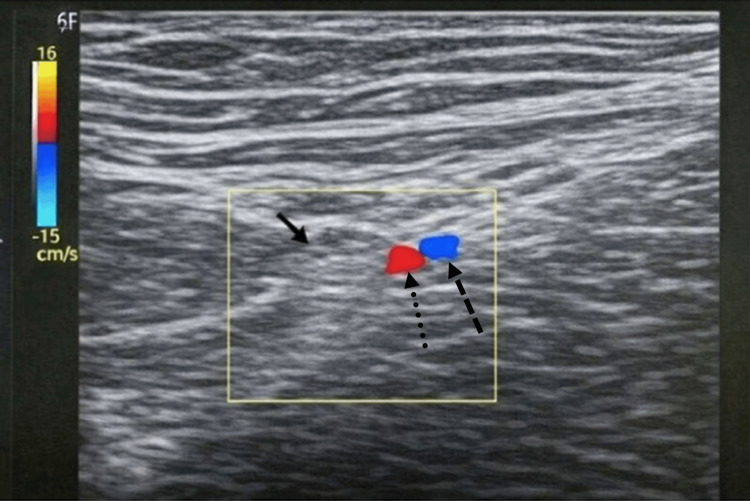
High-resolution short-axis ultrasound image of the right inguinal region demonstrating focal thickening of the genitofemoral nerve (arrow) adjacent to the inguinal ligament and inferior epigastric artery (dotted arrow) and vein (dashed arrow).
